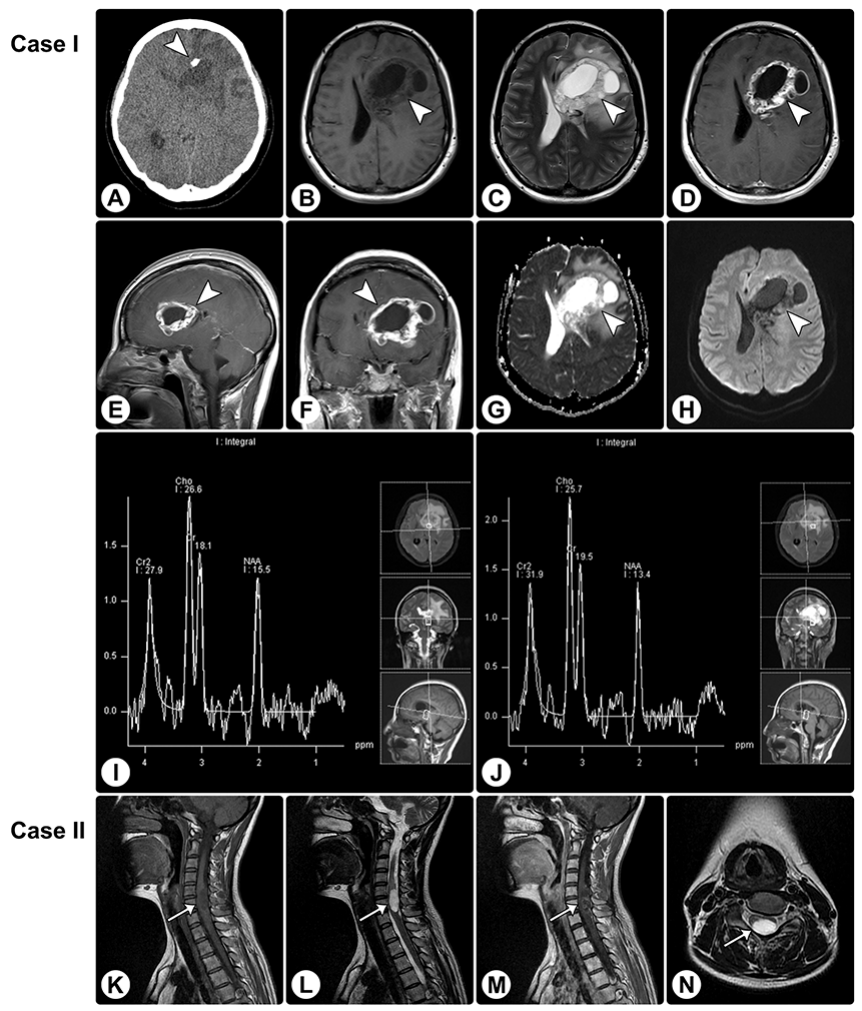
Rosette-forming glioneuronal tumor in the frontal lobe involving the lateral ventricle and rosette-forming glioneuronal tumor in the spinal cord (A) CT reveals a hypodense lesion (arrowhead) in the right frontal lobe involving the lateral ventricle, and focal calcification is visible. (B and C) MRI shows a cystic-sold lesion (arrowheads) with hypointensity on axial T1WI (B) and hyperintensity on axial T2WI (C). (D–F) The axial (D), sagittal (E), and coronal (F) contrast T1WI show heterogeneously remarkable enhancement in the solid portion of the tumor. (G and H) The apparent diffusion coefficient (ADC) map (G) and DWI (H) show facilitated diffusion. (I and J) MRS demonstrates an elevated choline value, reduced NAA value, and absence of lactate or lipid peaks. (K–M) MRI of another patient reveals an intramedullary mass (arrows) in the spinal cord, with hypointensity on sagittal T1WI (K), hyperintensity on sagittal (L) and axial (N) T2WI, and heterogeneous enhancement on sagittal contrasted T1WI (M).
